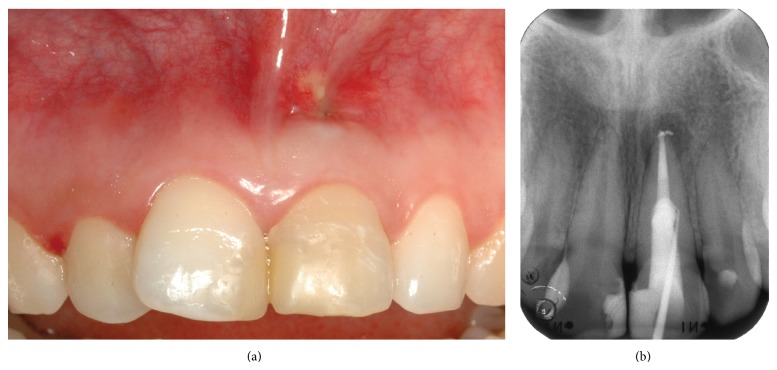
(a) Discoloration of the mucosa at the site of the perforation. (b) Radiograph showing the persistence of the coronal fistula.
